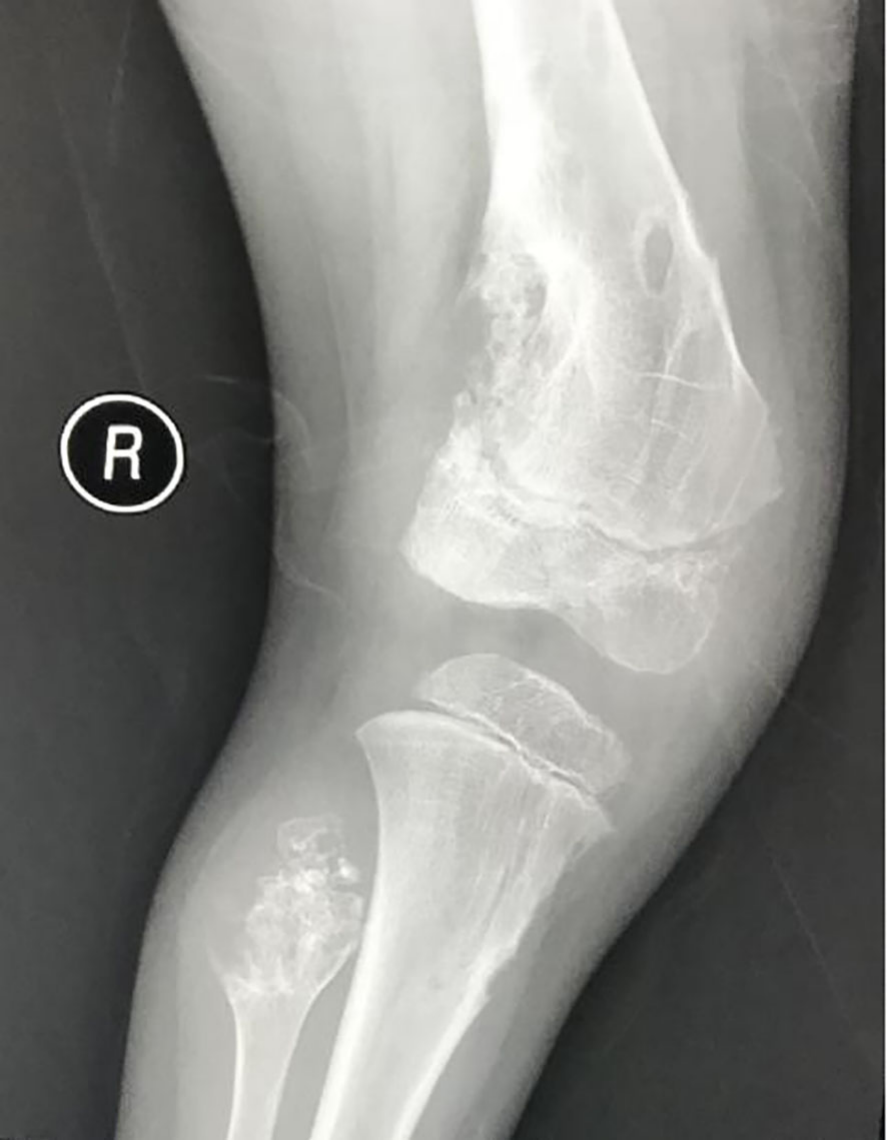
Plain bone X-ray showed multiple lytic bone lesions in distal part of the right femur, right tibia and fibula.
